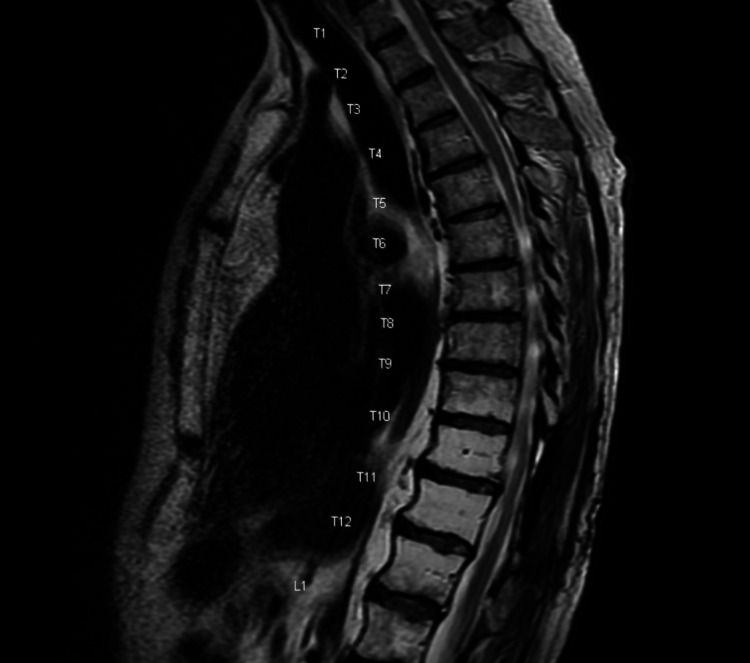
MRI of thoracic spine, T2 sagittal view. No evidence of disease recurrence.
